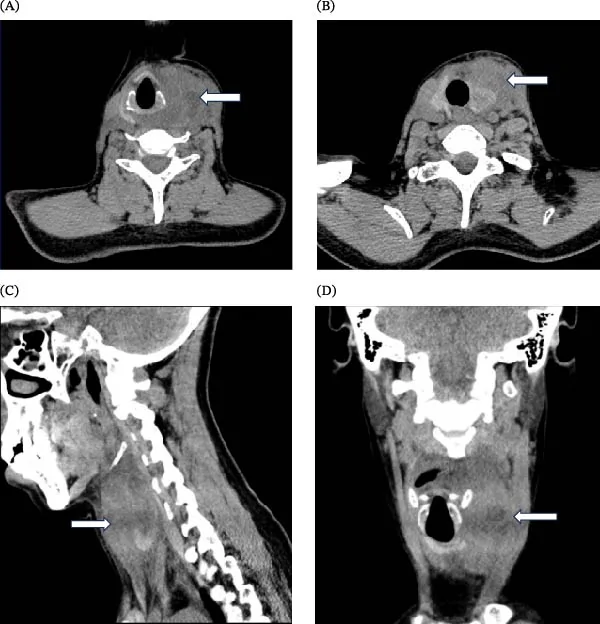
Non‐contrast CT images of the neck. (A) Axial CT showing a necrotic lesion of the left thyroid region with retropharyngeal extension and airway narrowing. (B) Axial CT demonstrating a low‐attenuation collection within the enlarged left thyroid lobe and adjacent esophageal edema. (C) Sagittal CT showing superior extension of the thyroid abscess above the hyoid bone. (D) Coronal CT demonstrating extensive left‐sided thyroid abscess formation with laryngeal edema and obliteration of the left pyriform sinus.
